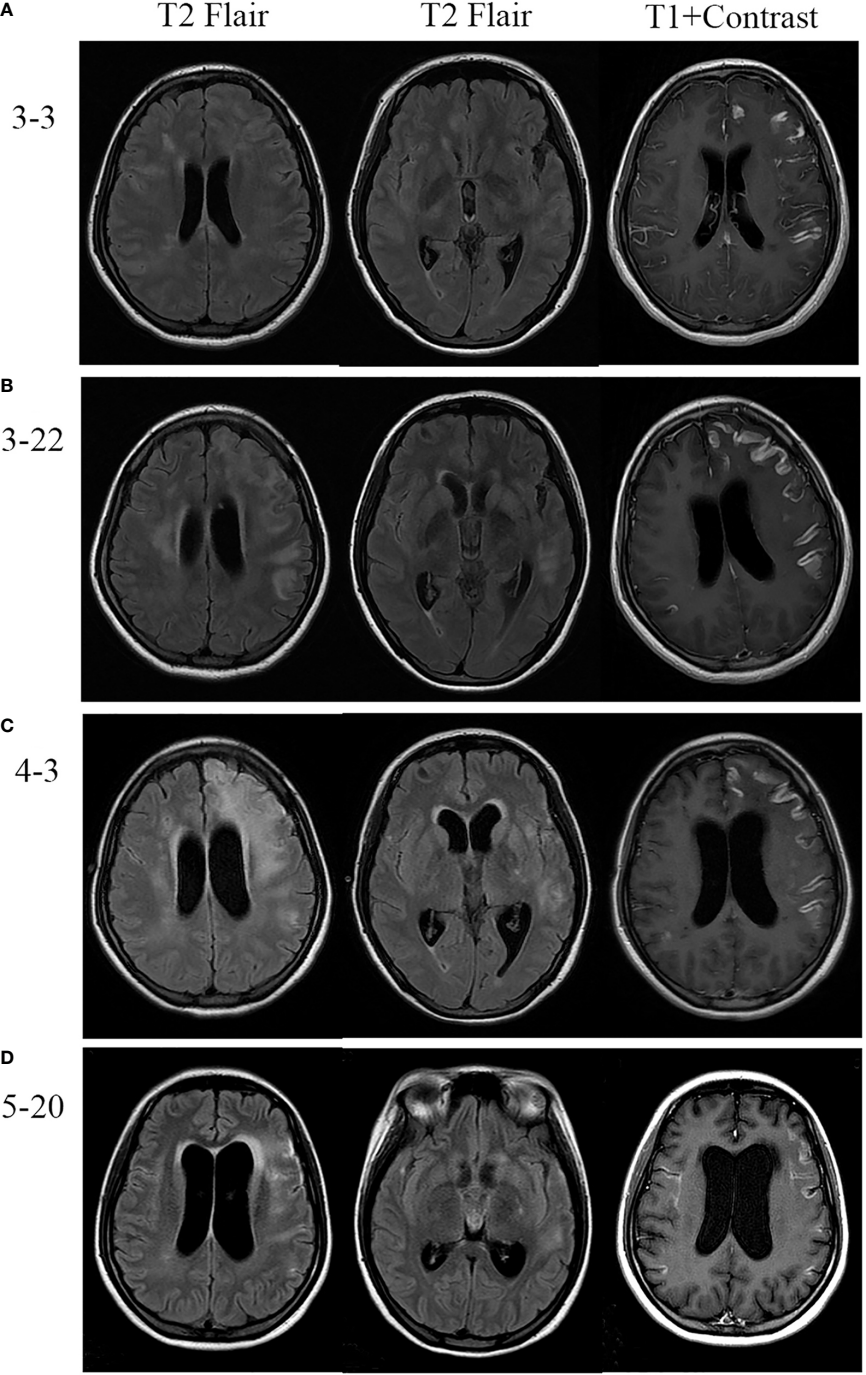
Brain MRI of the patient. (A) MRI revealed diffuse hyperintensities on fluid attenuated inversion recovery (FLAIR) imaging, accompanied by meningeal enhancement on the T1 contrast image. (B, C) Repeated MRI revealed that lesions were enlarged and meningeal enhancement was even more obvious after effective antibiotic therapy and induced labor. (D) Follow-up MRI showed marked improvement after corticosteroids treatment.
